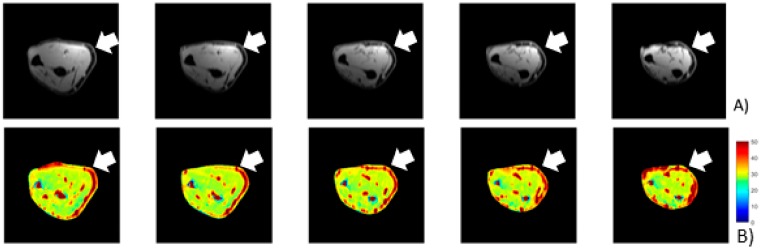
(A) Pretreatment of T1 MRI where the thickness of the brachial fascia is evident. The fascia is the black ring below the skin identified by the arrow. (B) Pretreatment T_1ρ_-mapping where the higher concentration of unbounded water is evident (water that it is not linked with any glycosaminoglycan or hyaluronan) within the brachial fascia in the symptomatic side (white arrow).
